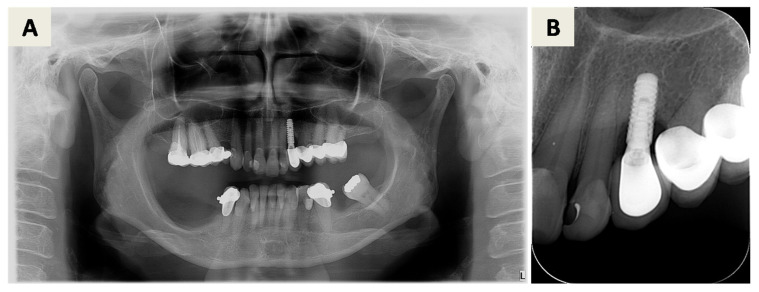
First radiographic examination. (A) Orthopantomogram and (B) RTG image showing visible peri-implantitis related bone defect around the implant in position 23 (FDI notation system).
